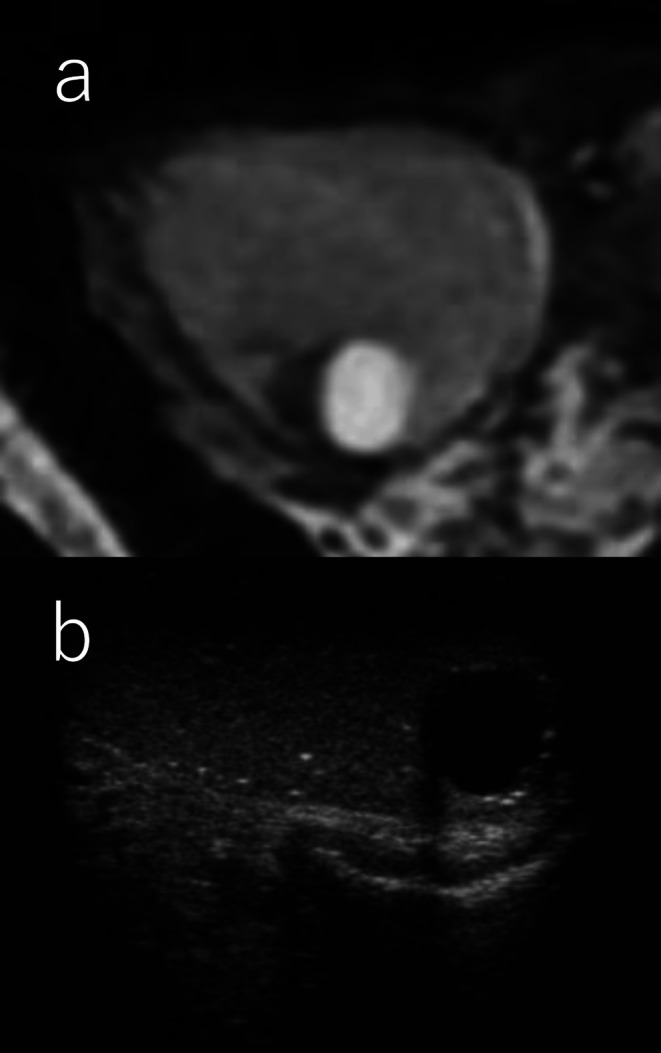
Magnetic Resonance Imaging and Ultrasonograms of bilateral testes at initial presentation. (a, b) Magnetic resonance imaging (MRI) and Ultra sounds (US) revealed only a 10‐mm simple cystic lesion in the right testis.
